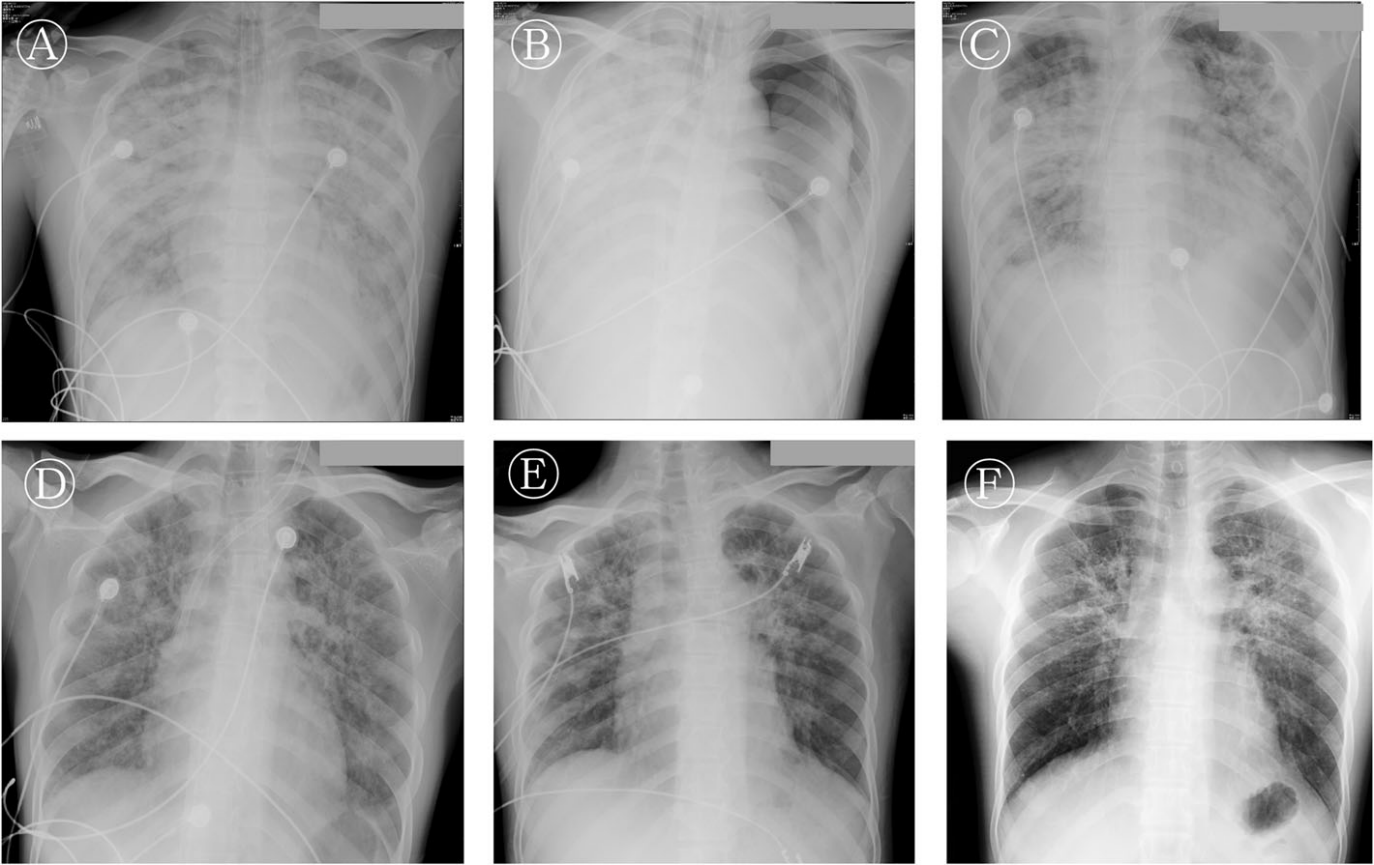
A typical serial chest radiographs of awake ECMO patients (Patient 10). a: On ICU admission day. This is the initial chest radiograph after intubation. Increased bilateral opacity in both lungs are noted. b: The second day after ICU admission. ECMO insertion was done due to refractory hypoxemia. Chest x-ray shows progressive increase of right opacity and newly left pneumothorax. The left pneumothorax was improved with chest tube and lower mechanical ventilation support level due to ECMO. c: On ECMO 5 days. There is improved pulmonary infiltration and pneumothorax, the patient was extubated and underwent awake ECMO to avoid ventilator-associated pneumonia. d: On ECMO 30 days. The patient was weaned from ECMO after this chest x-ray. e: Fourteen days after weaning from ECMO and discharge. f: one month after discharge
